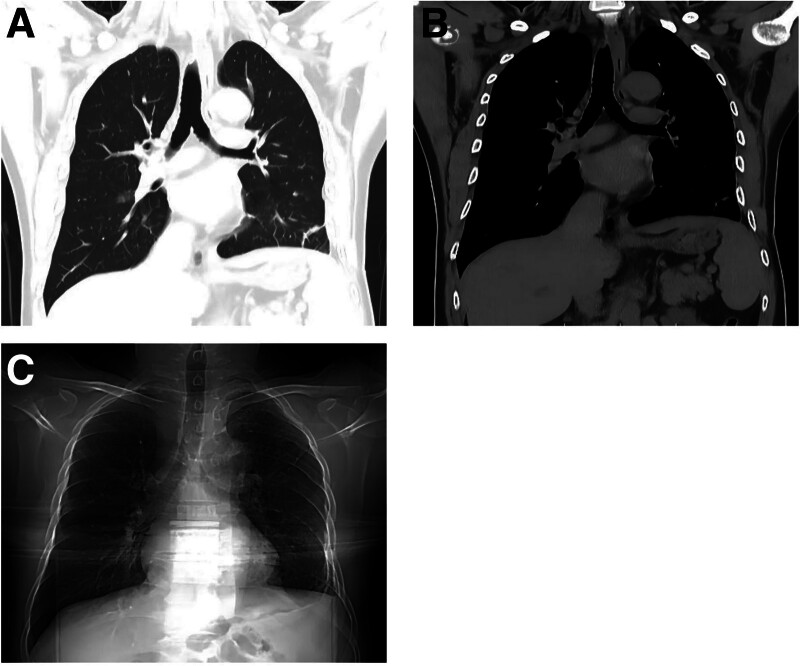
Follow-up imaging results. (A, B) Six months postoperatively, chest CT shows bilateral lungs essentially normal, with the liver, spleen, and stomach in their proper anatomical positions. (C) Two-year postoperative follow-up chest X-ray reveals no significant abnormalities. CT = computed tomography.
